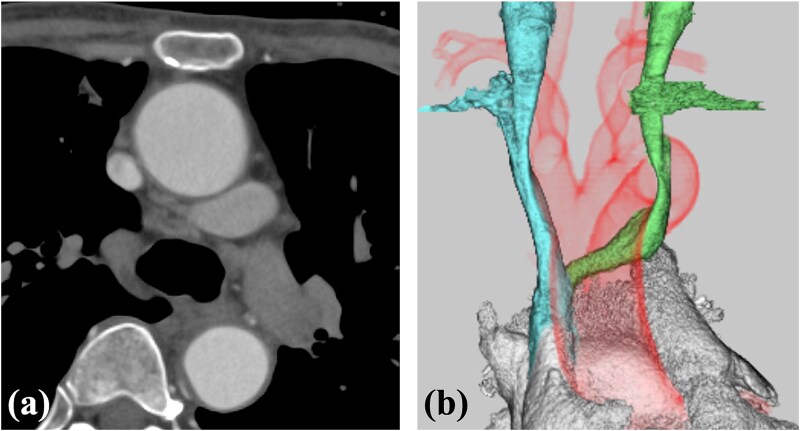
Contrast-enhanced CT. (a) The ALBCV coursed posterior to the ascending aorta. (b) The ALBCV joined the SVC at a peripheral location ⁓3 cm from the right atrium. No right aortic arch or double aortic arch was observed.
